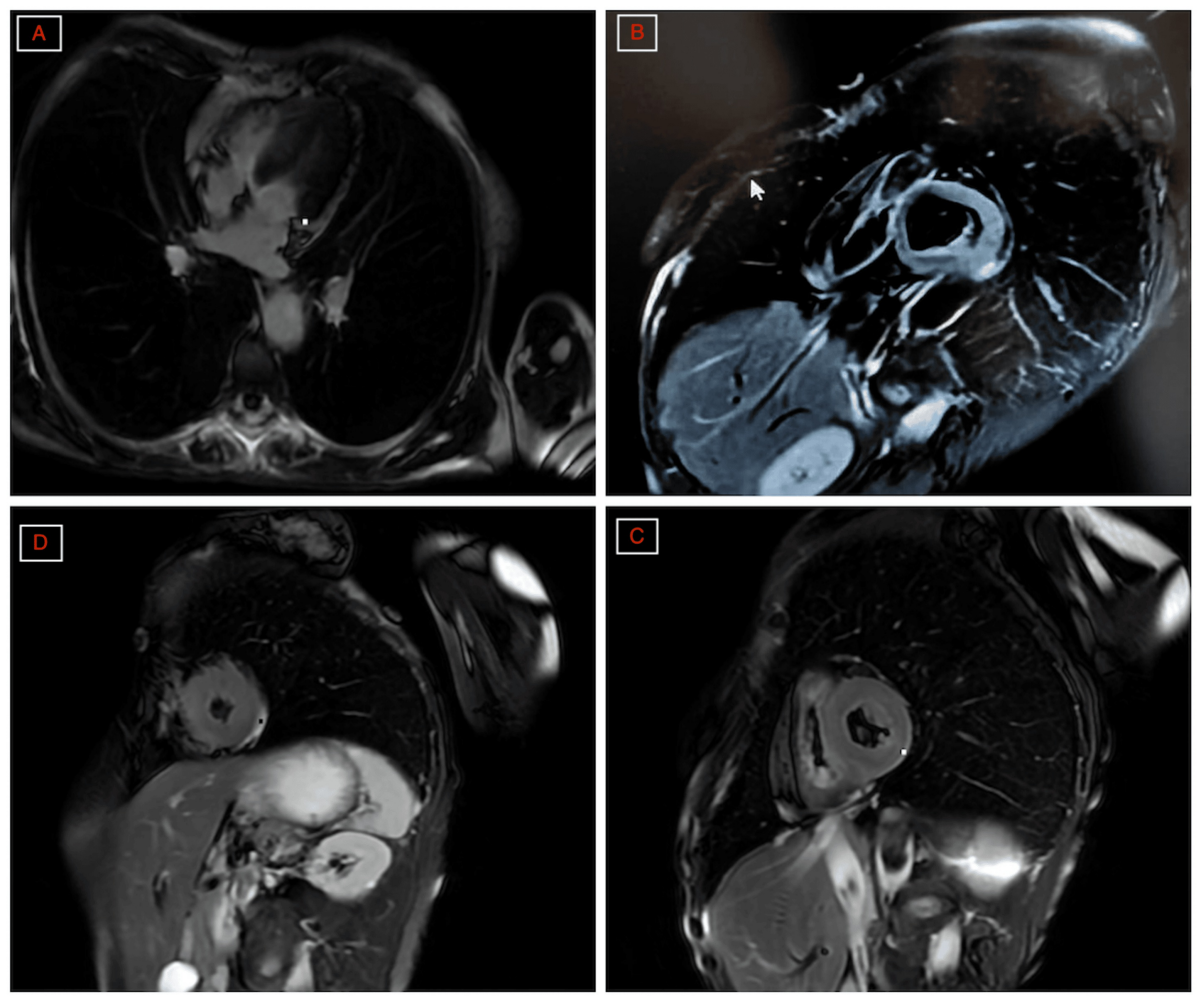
Cardiac magnetic resonance imaging findings on day 3. Cardiac magnetic resonance imaging findings on day 3. (A) Four-chamber late gadolinium enhancement (LGE) view showing no myocardial LGE. (B) T2-weighted black-blood fat-saturated short-axis image at the basal level, without significant myocardial edema. (C) T2-weighted black-blood fat-saturated short-axis image at the mid-ventricular level, showing mild myocardial edema. (D) T2-weighted black-blood fat-saturated short-axis image at the apical level, also showing mild myocardial edema.
